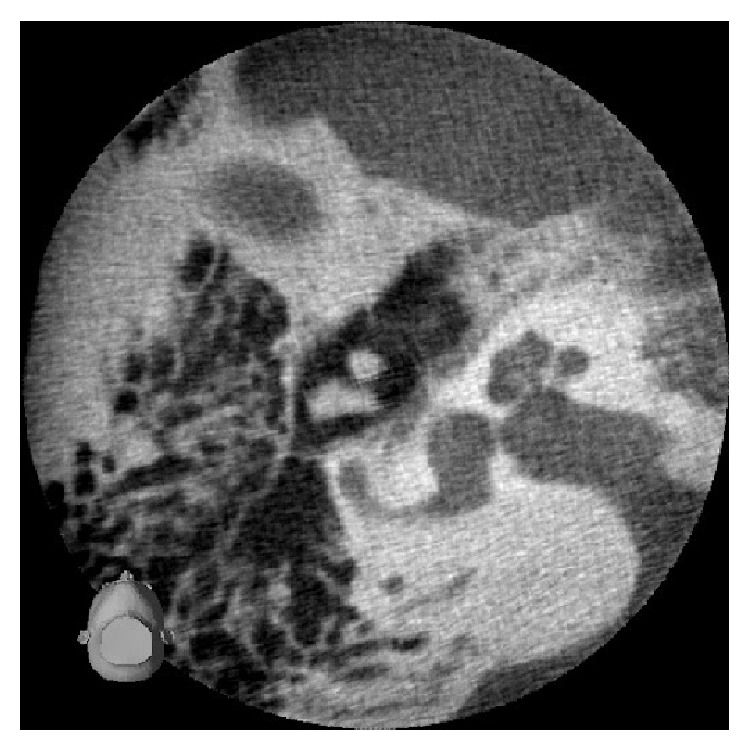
Axial view of the right ear on cone-beam computed tomography obtained 2 years after initial presentation. The air bubble apparent in Figure 2(a) had disappeared.
